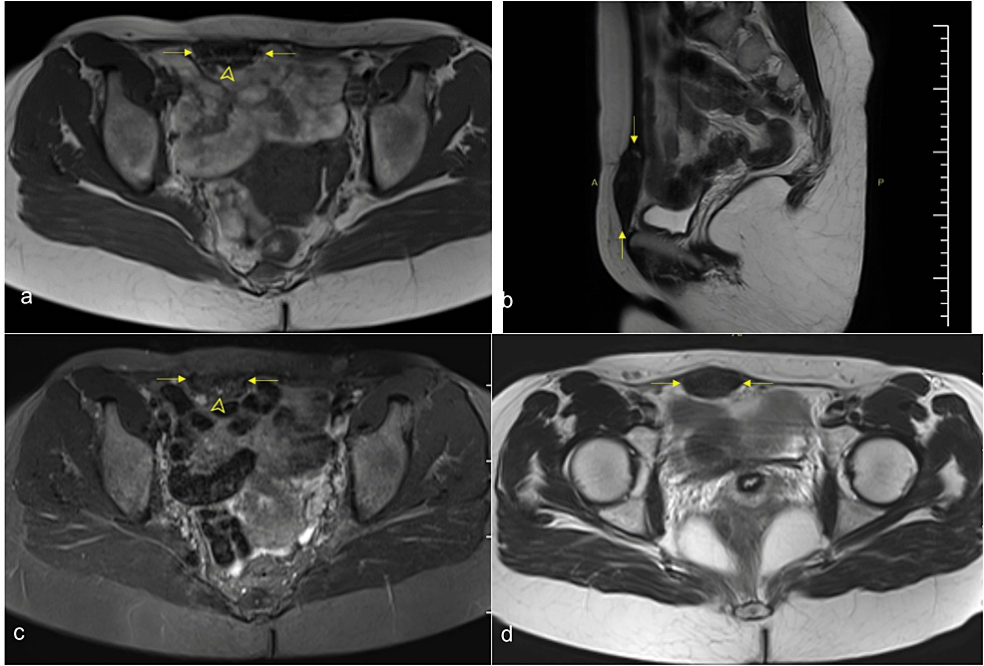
T1-weighted axial image (a), T2-weighted sagittal (b), and axial (d) images showed a hypointense lesion in the right rectus abdominis muscle (arrow). On the axial STIR sequence (c), which was at the same level of the image (a) a high internal punctate signal intensity became more conspicuous (arrowhead). STIR: short T1 inversion recovery.
